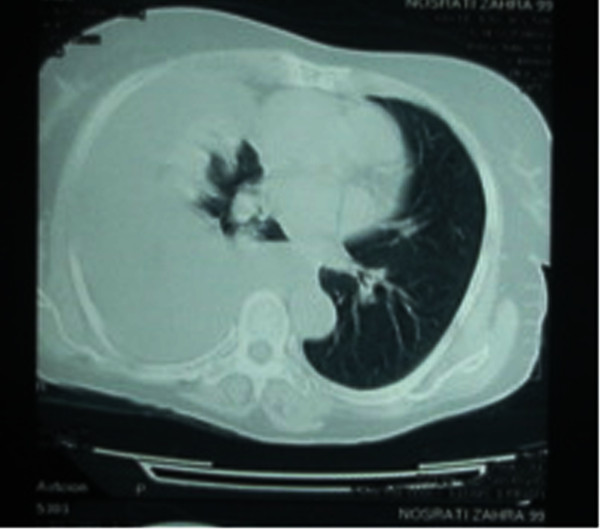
Chest CT scan of the patient demonstrating pleural effusion.
